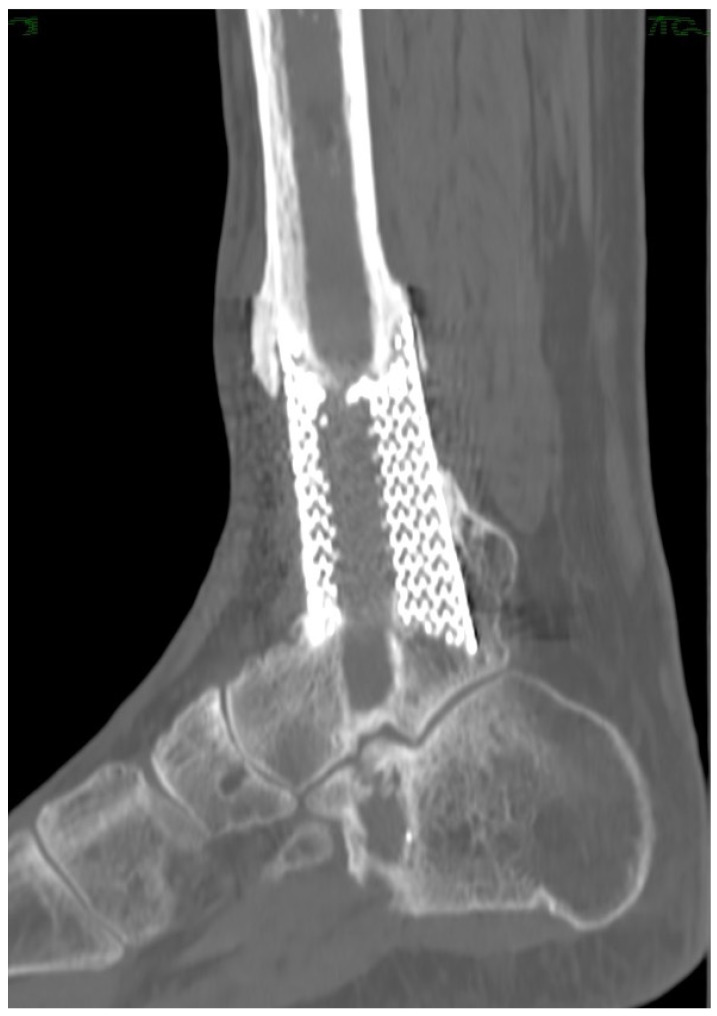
CT scan at final follow-up, after intramedullary nail removal. Note the proximal and distal osteointegration of the custom-made implant.
